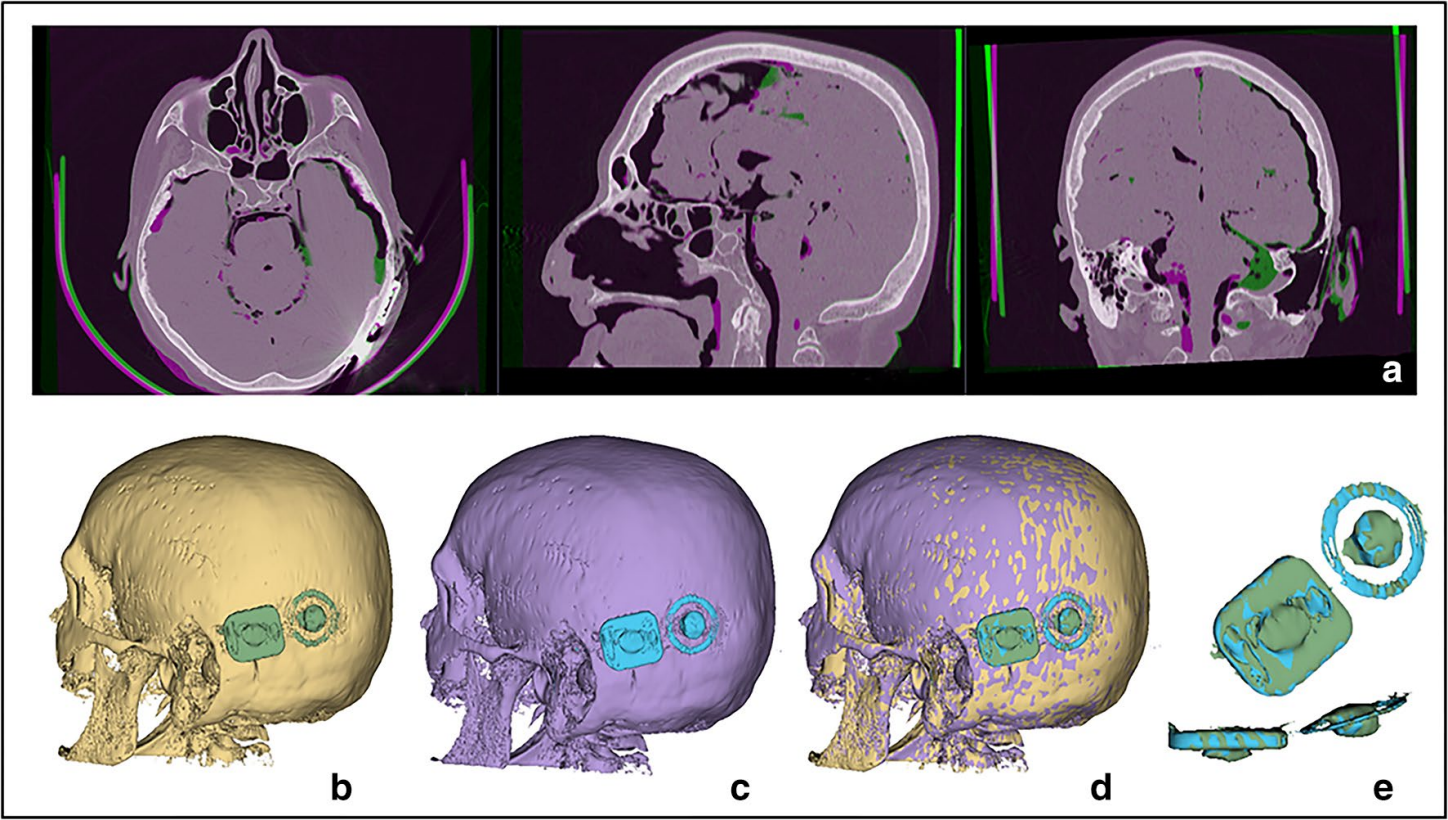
CI displacement analysis. a Co-registration of high-resolution CTs before and after MRI, both with CI. Green or purple areas show differences between the two images and are mainly limited to some displacement of soft tissue (brain). Bone structures are rigidly co-registered and do not show any signs of displacement. On the axial view, the device is already visible on both pre- and post- MRI without any signs of repositioning. b–e Assessment of CI displacement in 3D reconstruction: b 3D head reconstruction before MRI (gray: skull; green: CI), c 3D head reconstruction after MRI (purple: skull; cyan: CI), d 3D head reconstruction before (gray: skull; green: CI) and after MRI (purple: skull; cyan: CI) to verify potential repositioning: no displacement was recorded, e particular of 3D CI reconstruction before (green) and after (cyan) MRI with full CIs concordance
